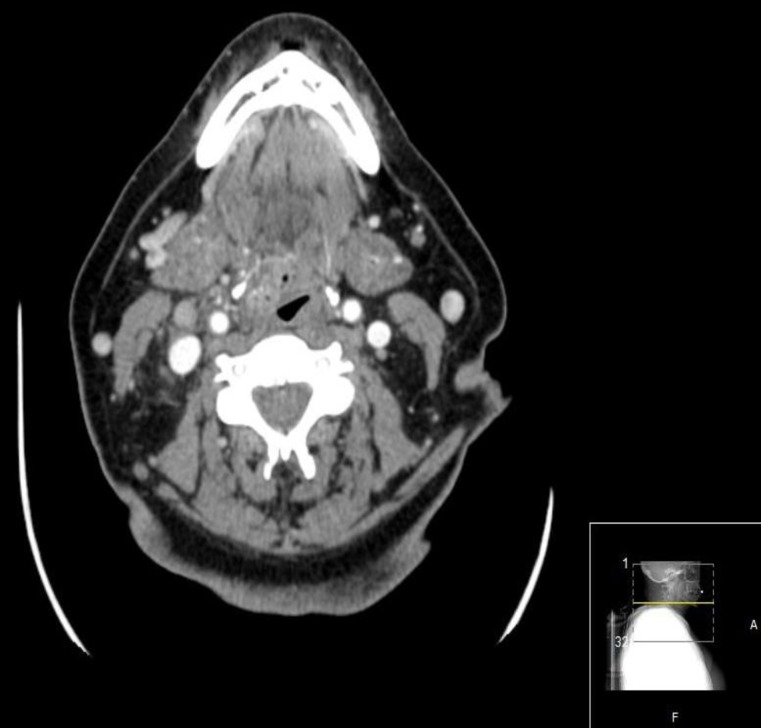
Cervical CT scan with a mass in the right vallecula contacting with the lateral posterior part of the tongue and partially obliterating the lumen of the aero-digestive way
